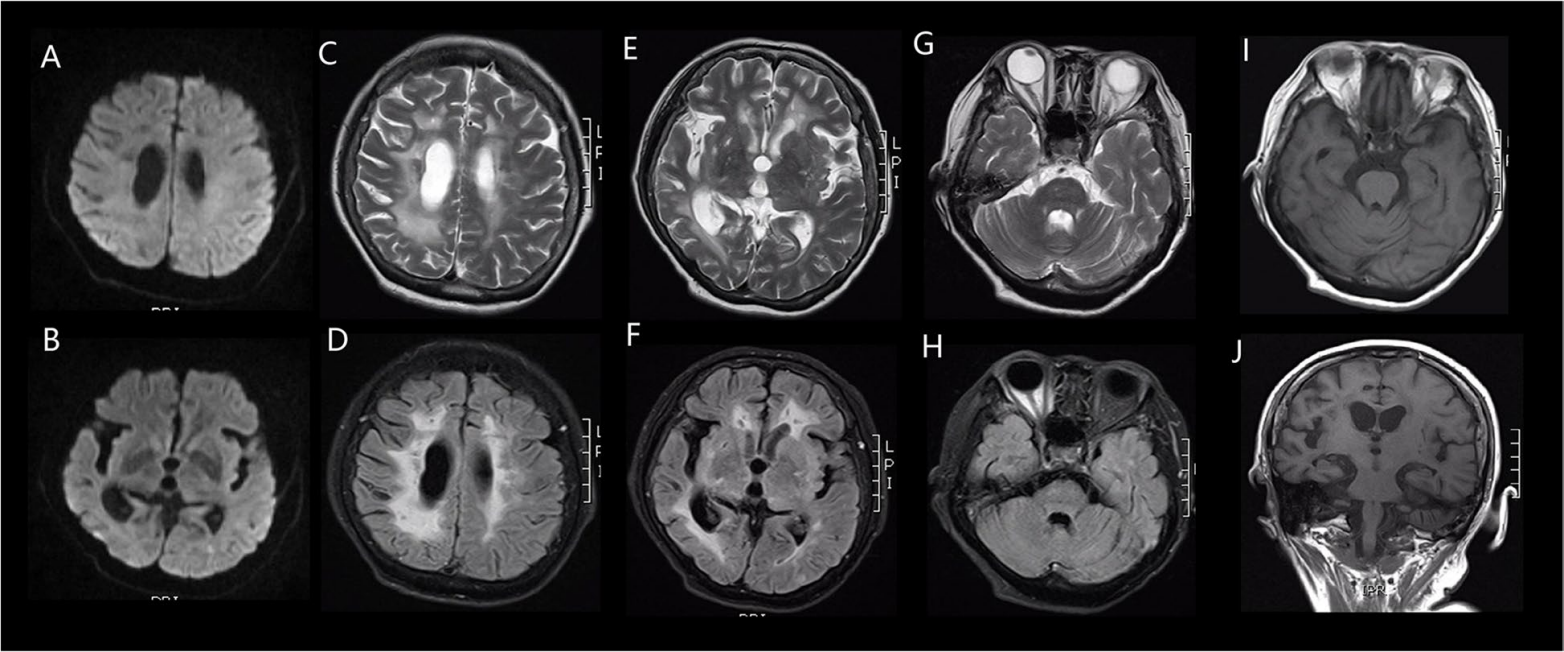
Brain MRI of the patient in November 2019 DWI showed no recent infarctions (A, B). MRI showed high T2 (C,E,G) and FLAIR (D, F, H) signals of multiple cortical and subcortical ischemic infarcts, softening foci, and extensive confluent white matter lesions. There is also global atrophy, particularly in the bilateral hippocampus (I, J) and the right temporo-apical lobe (C-F)
